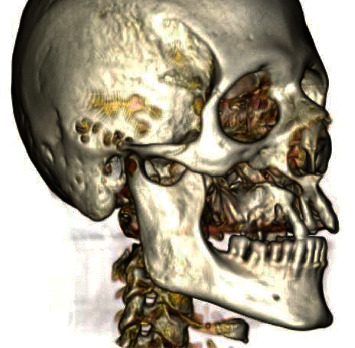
Three-dimensional reconstruction of CT scans that highlighted the detachment of the right hemimaxilla.
